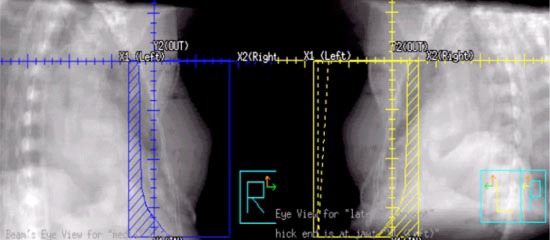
(Color) Lateral and medical tangent digitally reconstructed radiographs for setup verification of the lateral and medial tangent treatment field. The heel of lthe wedge is towards the $X_{1}$ (left) yaw as indicated in the DDR of the lateral tangent field on the right.
